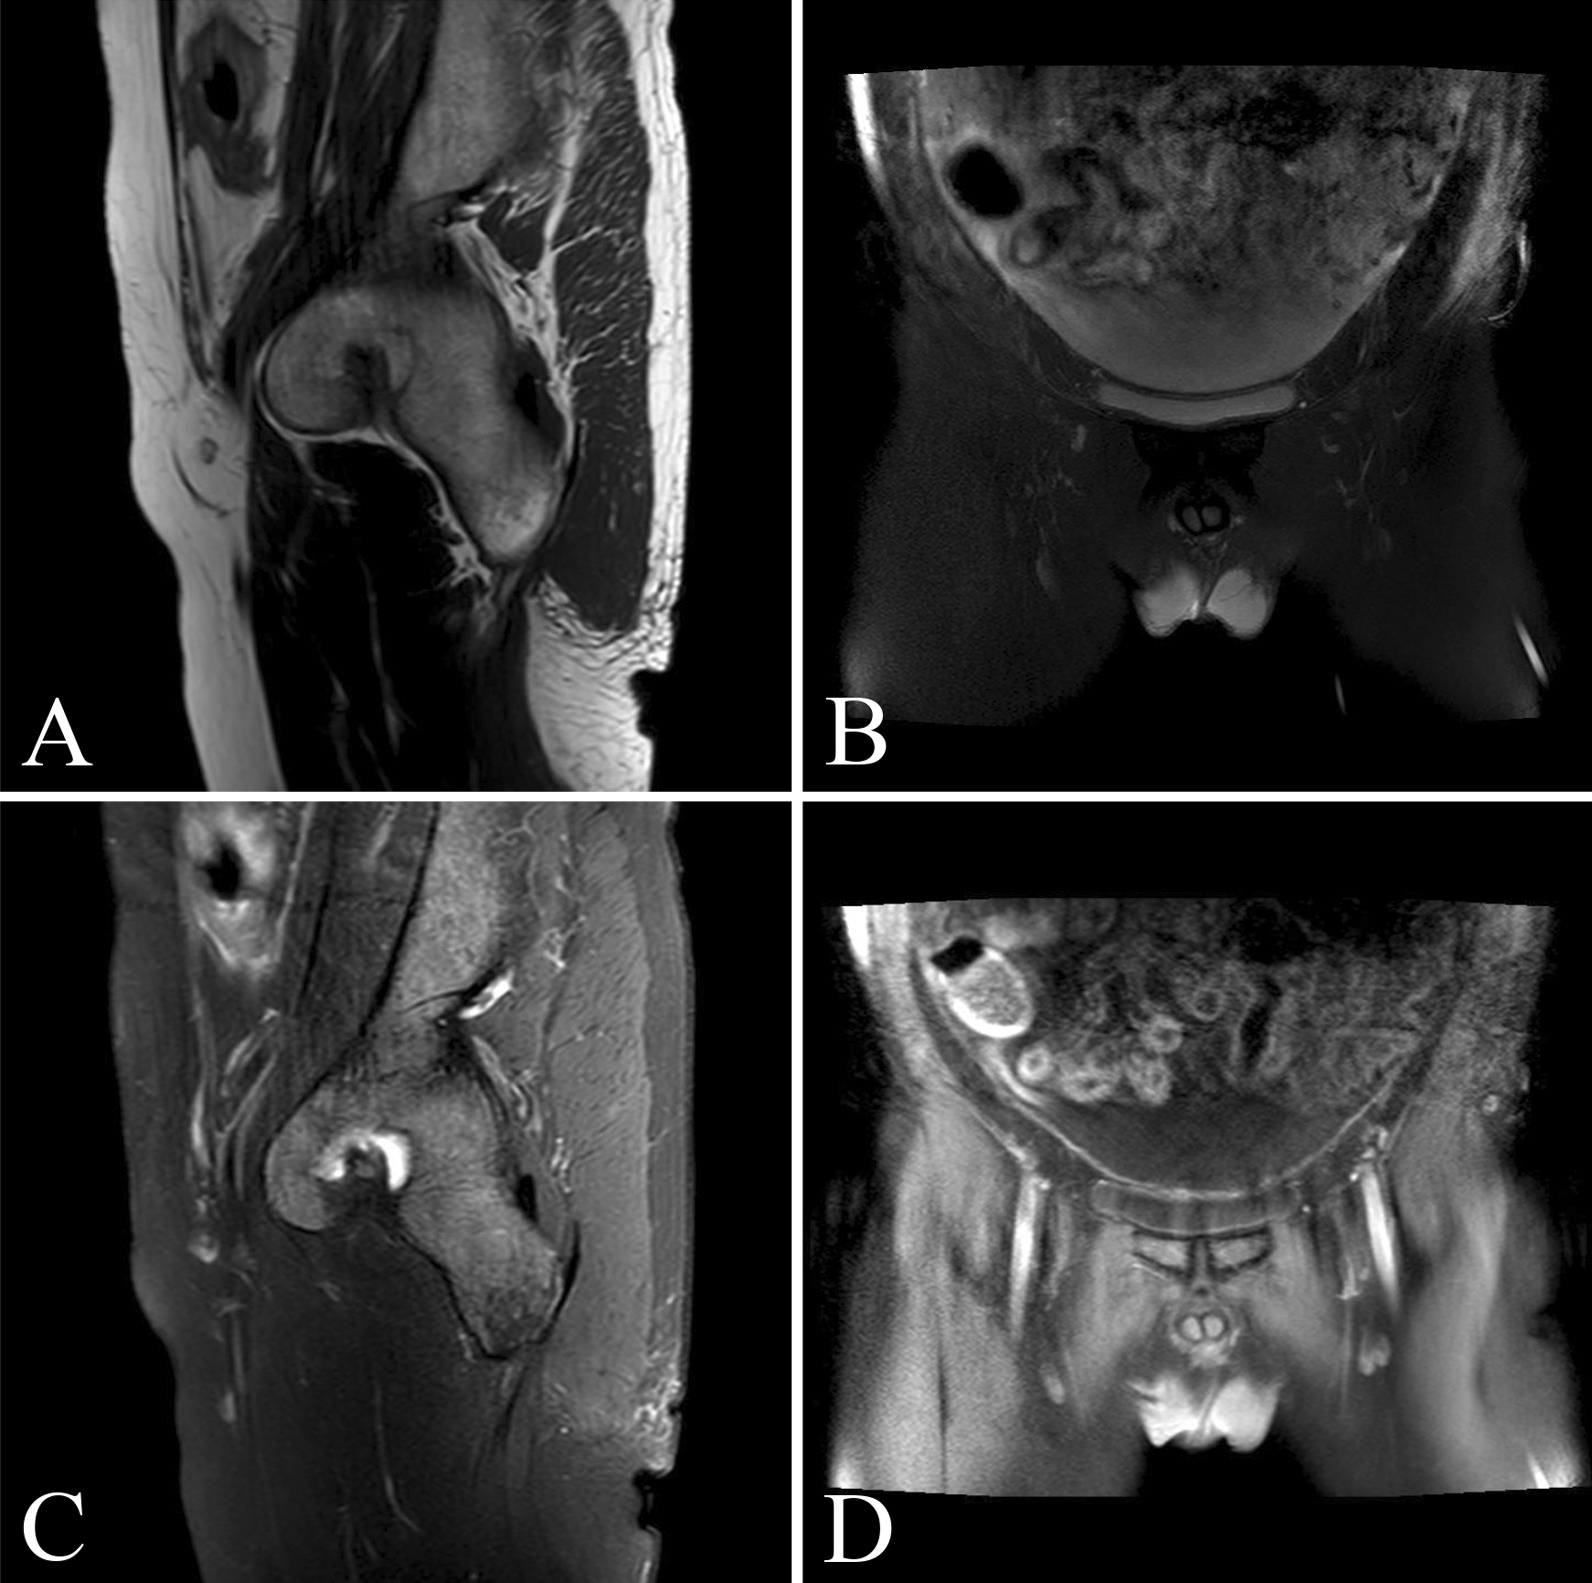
Magnetic resonance images of lower abdomen and pelvis. MRI showing the local intestinal wall of sigmoid colon was thickened (a, c), the peritoneum was unevenly thickened, and there was liquid signal shadow in pelvic cavity (b, d)
